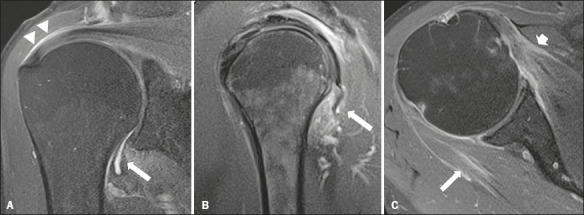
MRI of the right shoulder in a 60-year-old female with PMR. A: Coronal
